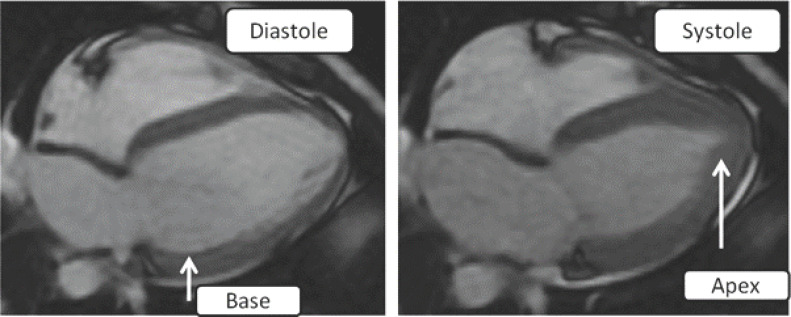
L2DStrain IRM cardiaque montrant une dysfonction systolique du ventricule gauche prédominante au niveau des segments basaux avec une bonne contractilité apicale Cardiac MRI showing predominant left ventricular systolic dysfunction in the basal segments with good apical contractility
